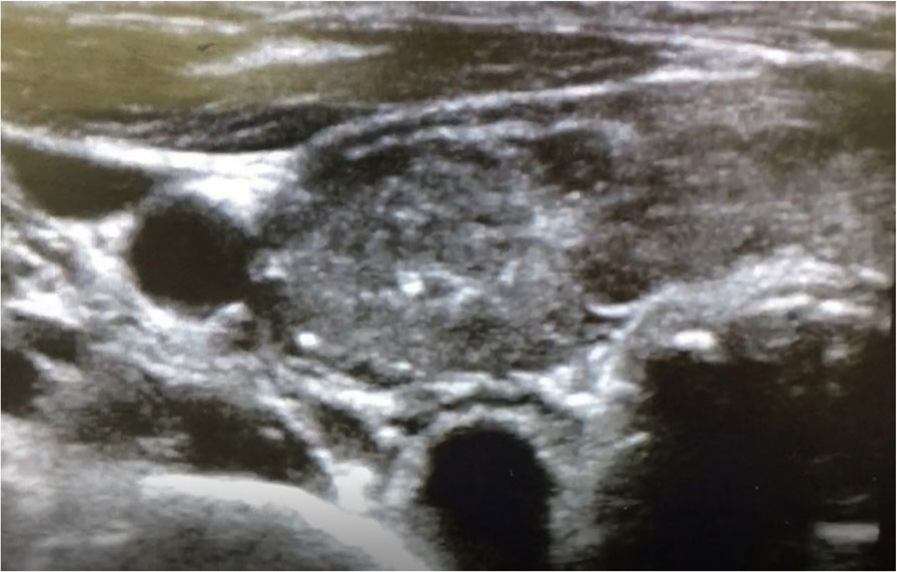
Ultrasonography of the thyroid. The left lobe is heterogeneously enlarged with hypoechoic areas and calcifications
